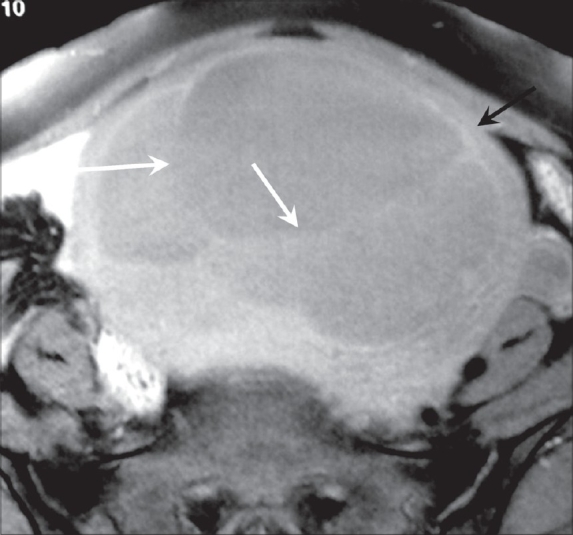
Fat-suppressed axial T1W MRI of the pelvis reveals a well-demarcated, thick-walled mass (black arrow), with the internal contents showing hypointense signal and multiple internal septae (white arrows)
